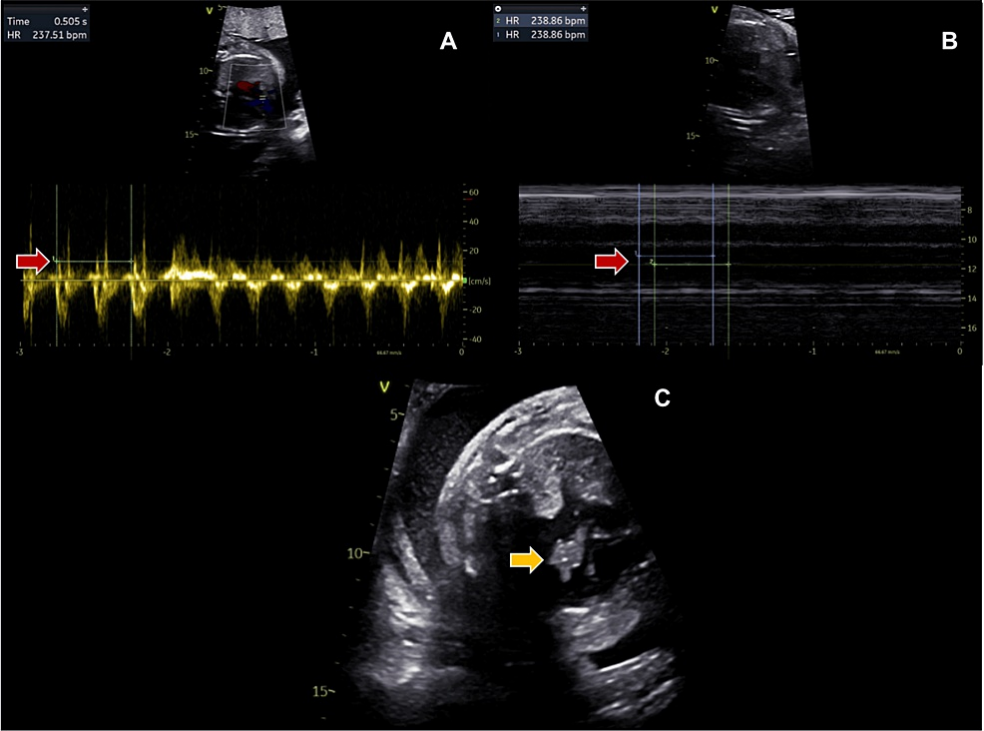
Fetal echocardiography image at 28 weeks of gestation. A) Color Doppler imaging captures blood flow through the fetal aortic valve, allowing for the measurement of the fetal heart rate. As indicated by the red arrow, in this case, it is 238 bpm. B) M-mode imaging slice captures the atrium and ventricle of the fetal heart, indicating a one-to-one relationship between atrial and ventricular contractions, showing normal electrical conduction from the atria to the ventricles (1:1 atrioventricular conduction). The heart rate is measured at 238 bpm, highlighted by the red arrow. These findings are consistent with supraventricular tachycardia. C) 2D imaging shows a homogeneous mass on the anterior leaflet of the mitral valve, measuring approximately 7x8 mm, indicated by the yellow arrow.
